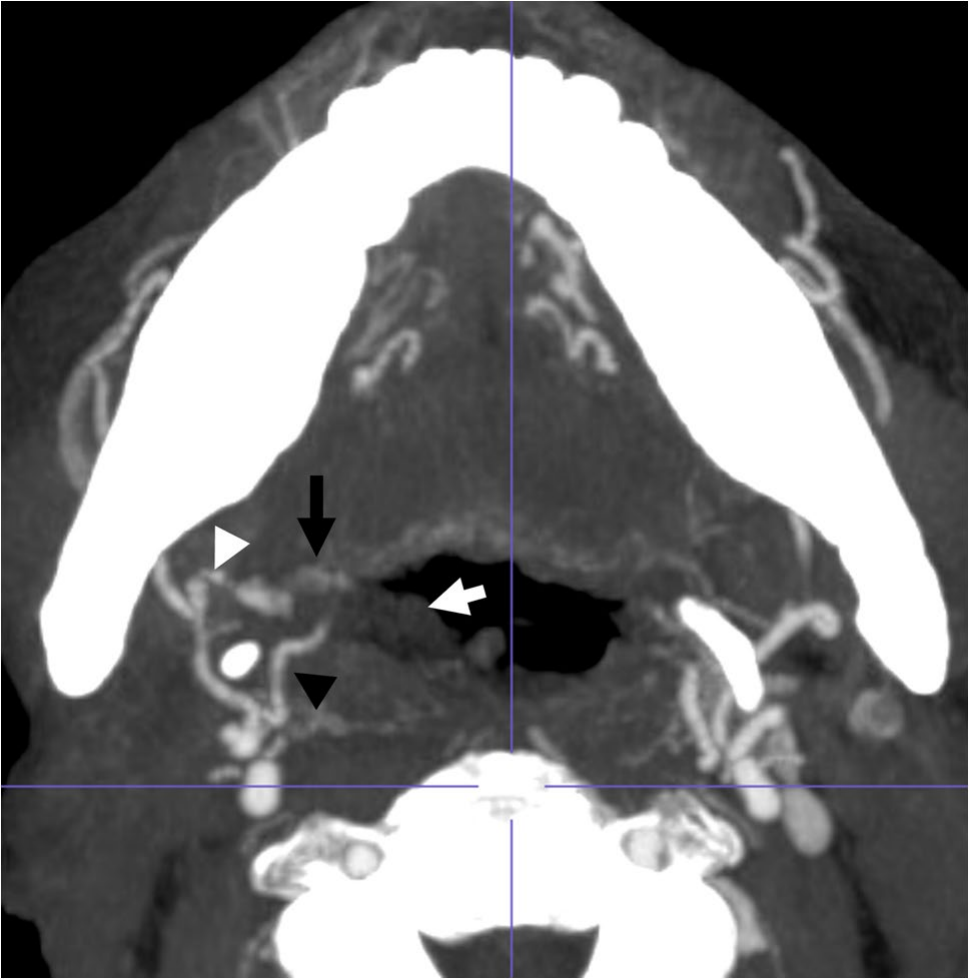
Patient 1. Axial postcontrast CTA MIP image of the neck at the level of the base of tongue demonstrates linear high-density contrast material in the right oropharyngeal lumen (black arrow) surrounded by low-density foamy debris (white arrow), appearing contiguous with a right facial artery branch (possibly ascending palatine or tonsillar) demonstrating irregular caliber changes (white arrowhead). The right lingual artery also appears to course into the intraluminal debris (black arrowhead)
